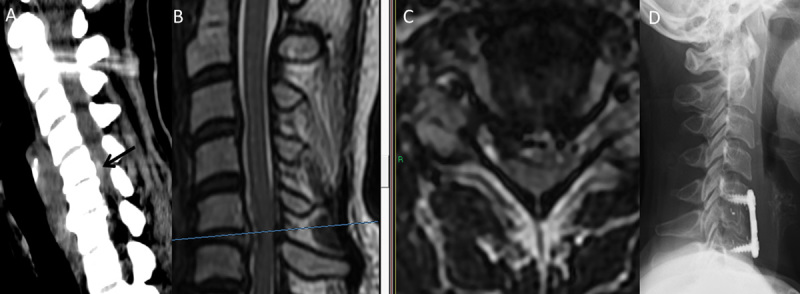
(A) Noncontrast admission computed tomography (CT) scan demonstrates C5–C6 disk herniation with canal stenosis (arrow) in a 47-year-old woman. (B) T2-weighted sagittal and (C) axial magnetic resonance imaging (MRI) scans performed 2 days later after neurologic deterioration reveal C5–C6 disk herniation with a hyperintense signal in the cord. (D) Postoperative lateral X-ray demonstrates a polyetheretherketone cage substitute for C6 vertebral body and anterior titanium plate.
